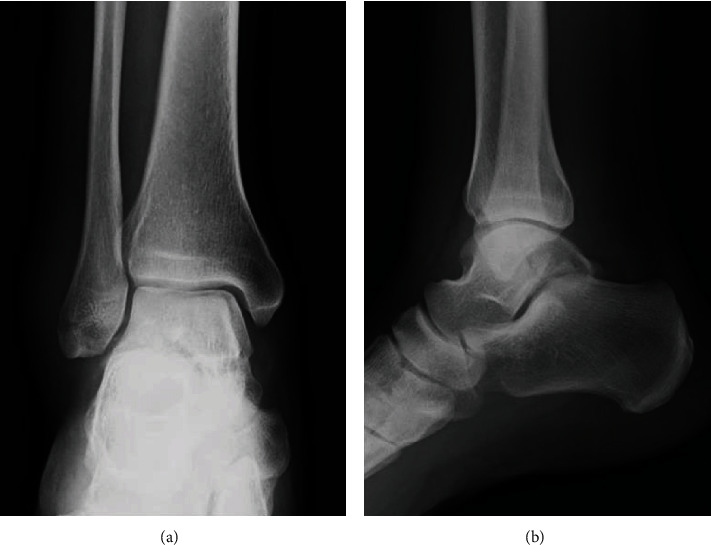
Plain radiographs of the right ankle showed no abnormal findings in (a) anteroposterior and (b) lateral views.
